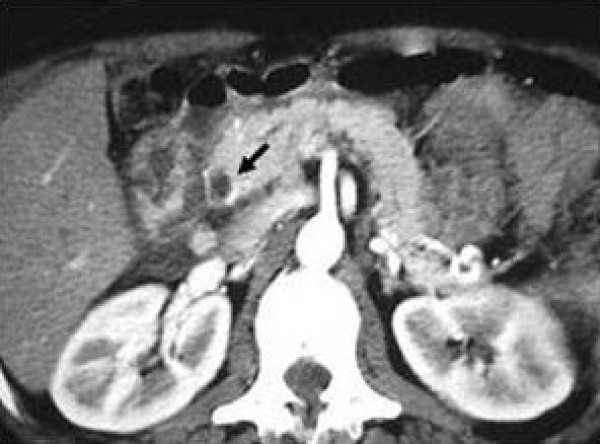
Computed Tomography scan after four months in case 1. Lesion found in the head of the pancreas (arrowhead).
